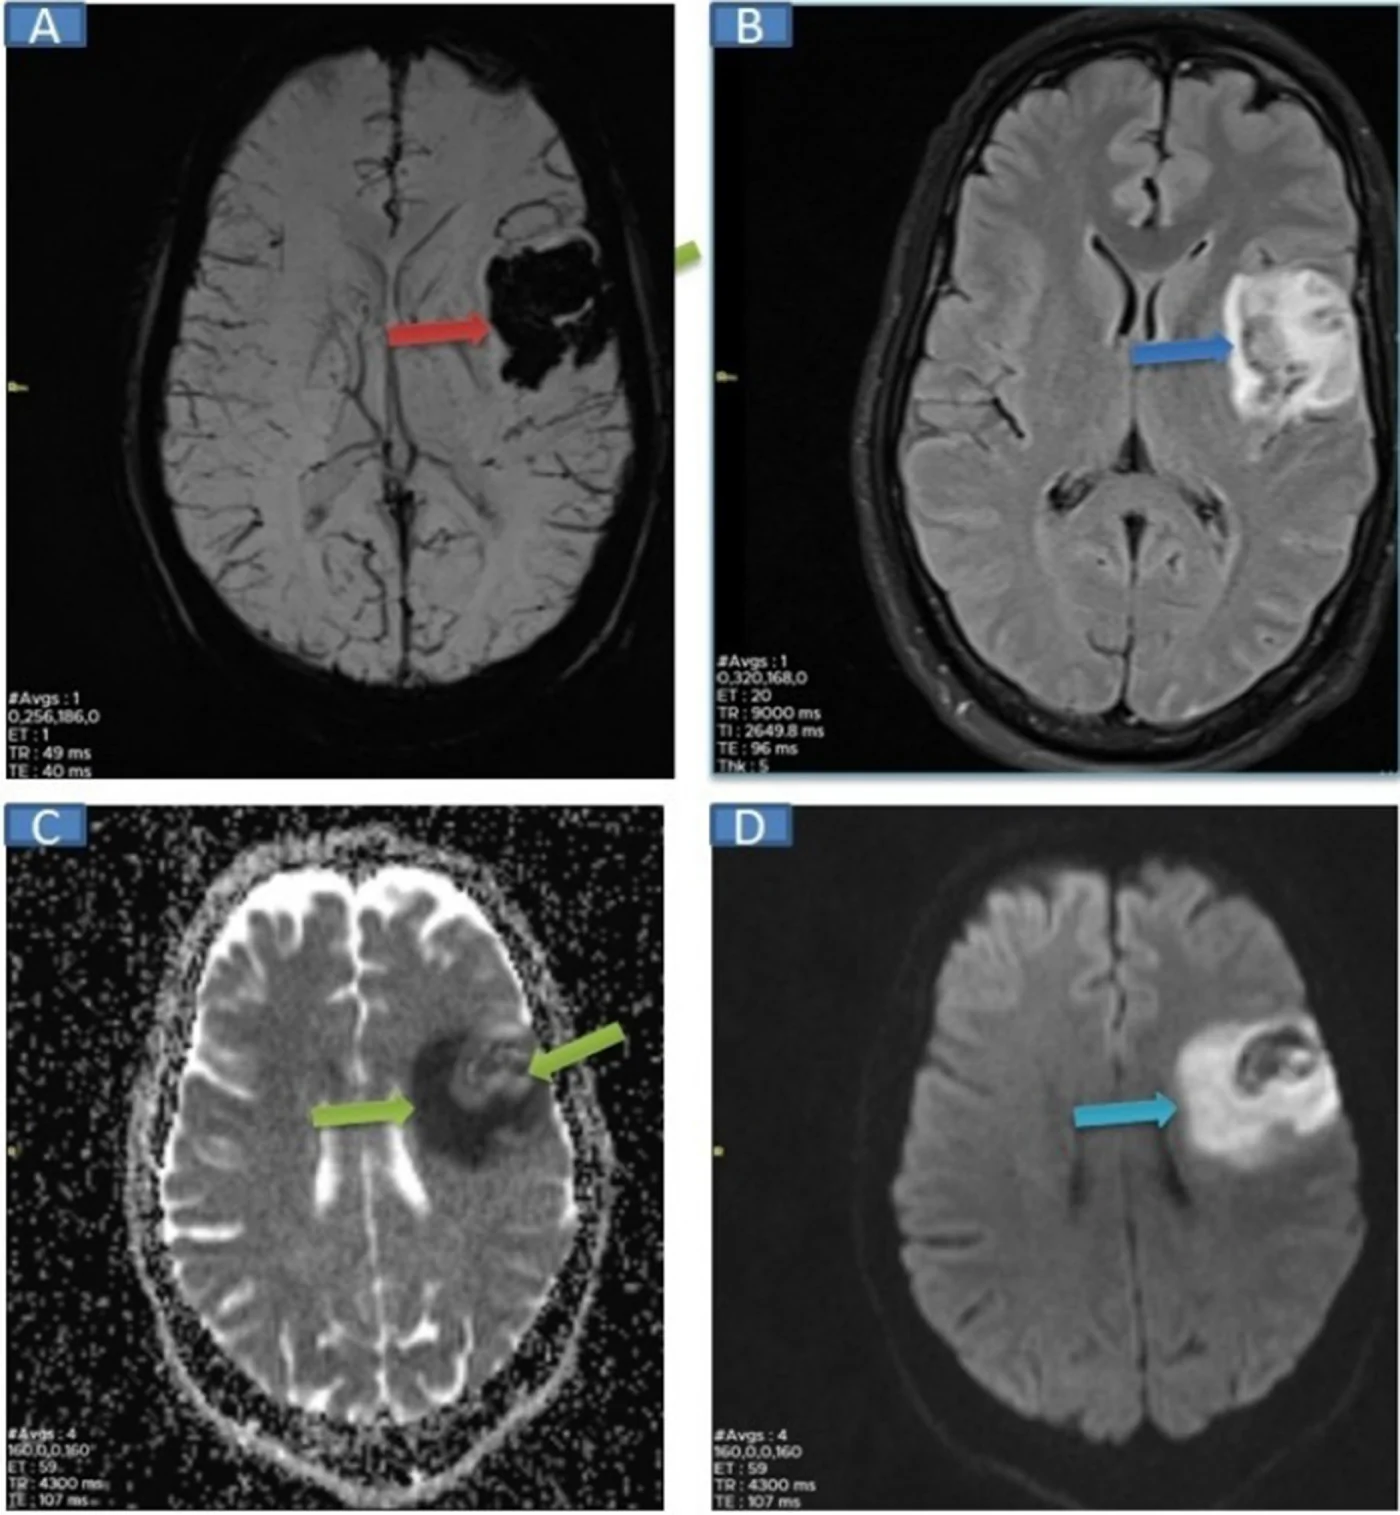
Imaging of left parietotemporal hemorrhagic ischemia. SWI shows blooming artifacts (red arrow), FLAIR demonstrates hyperintense signal (blue arrow), DWI reveals high signal intensity (green arrow), and the ADC map shows a corresponding low signal (light blue arrow).
